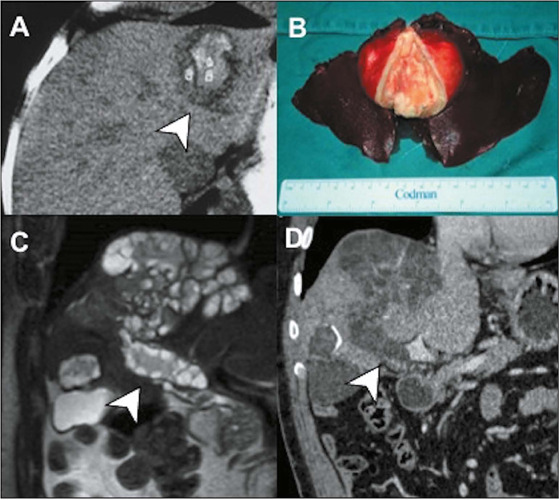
Types III and IV hydatid cysts. Unenhanced CT scan
